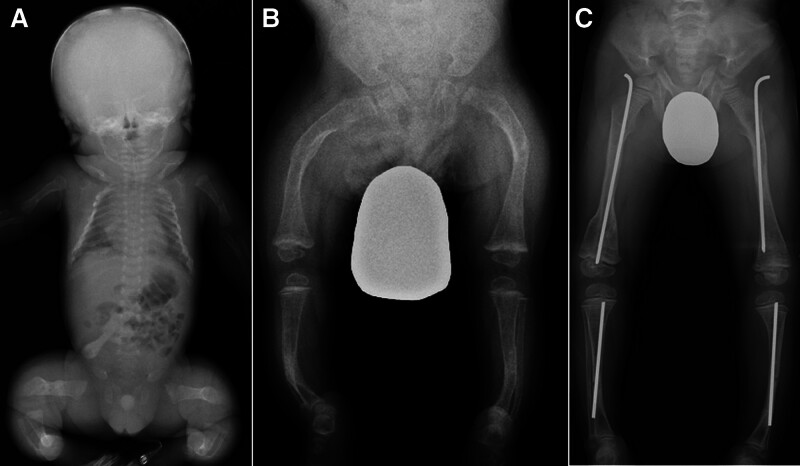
Serial radiographs of a 6-year-old boy with osteogenesis imperfecta. A neonatal babygram demonstrating multiple rib and long-bone fractures of the upper and lower extremities (A) The patient’s birth height was 36.0 cm (−6.2 SD). A preoperative anteroposterior radiograph of the lower extremities at the age of 2.7 years showing bowing of the femora and tibiae with decreased bone density (B). His height immediately before the first surgery was 73.4 cm (−6.6 SD). An anteroposterior radiograph of the lower extremities at the age of 6.0 years showing bilateral intramedullary rodding for the femora and tibiae (C). He has not currently acquired practical walking ability.
